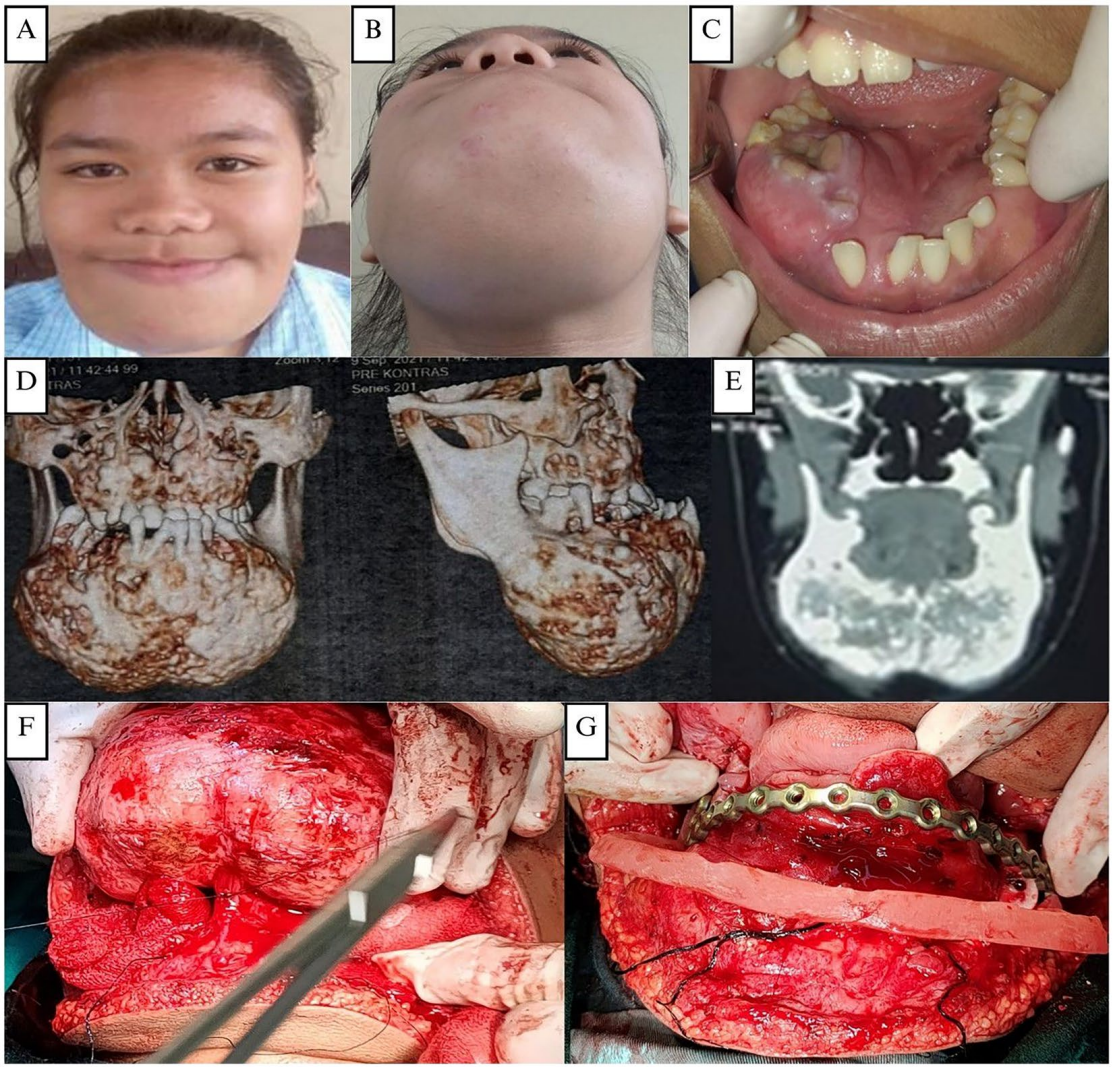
(A and B) The fourth case, a 15-year-old adult female patient with swelling in the right mandibular area. (C) Pre-operative intraoral clinical appearance. (D–E) CT scan showing an enlargement in the right ramus mandibular area with the typical ‘ground glass’ like opacification of the lesional bone, which blended with the adjacent normal bone. CT scan showing the size of the right mandibular body is larger than the left, no bone erosion and periosteal reaction is seen. (F–G) Surgical exposure, bone reshaping, and layers of closure of the right mandibular intraoral area.
